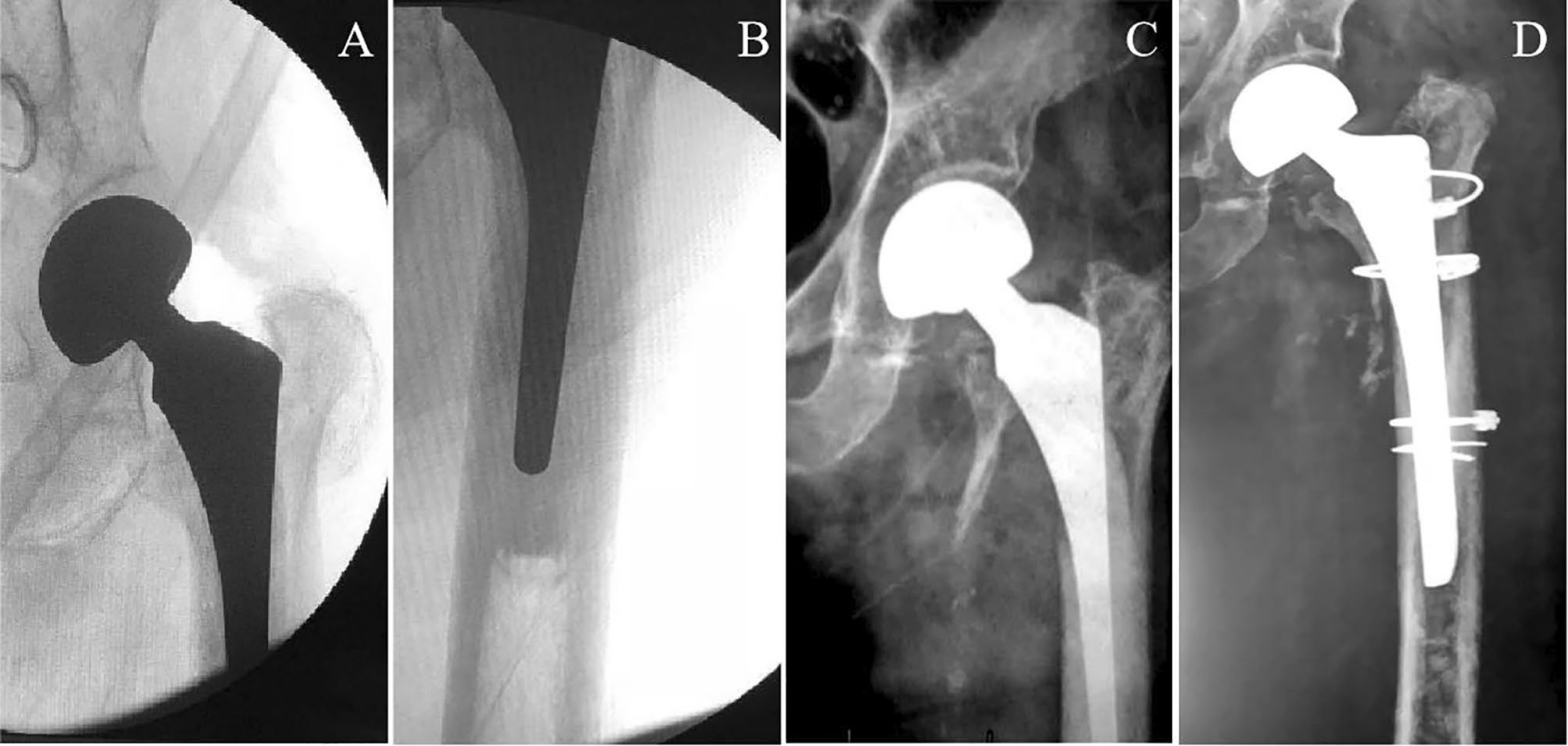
Case 1, an 82-year-old man underwent left hemi-arthroplasty with a cemented stem because of a left femoral neck fracture. (A–B) Two intraoperative radiographs taken with a C-arm machine. (C) After a fall, the continuity of the left medial femoral cortex was interrupted on an anterior–posterior radiograph. (D) Postoperative anterior–posterior radiograph after longer stem revision and internal fixation with cables.
